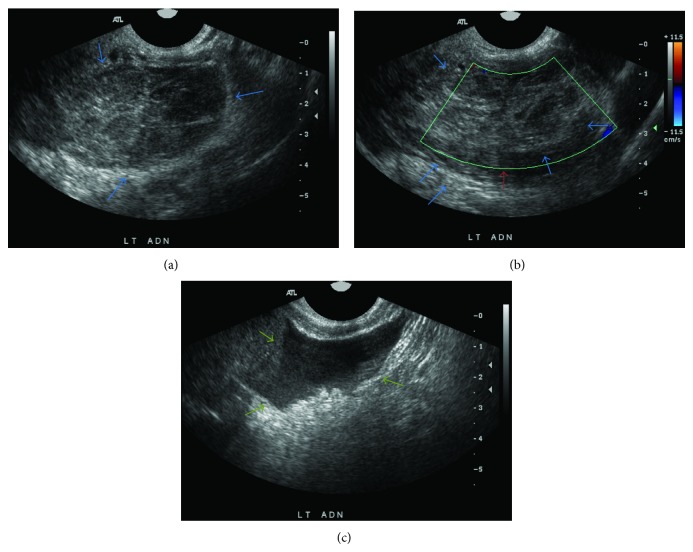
Sonographic images of the left adnexal region with and without color Doppler: large left adnexal mass (blue arrows), measuring approximately 6.3 × 3.9 cm, with heterogeneous echogenicity and no appreciable flow on color Doppler (red arrows). There is a moderate amount of free fluid (green arrows). Left ovary not definitively visualized [3].
